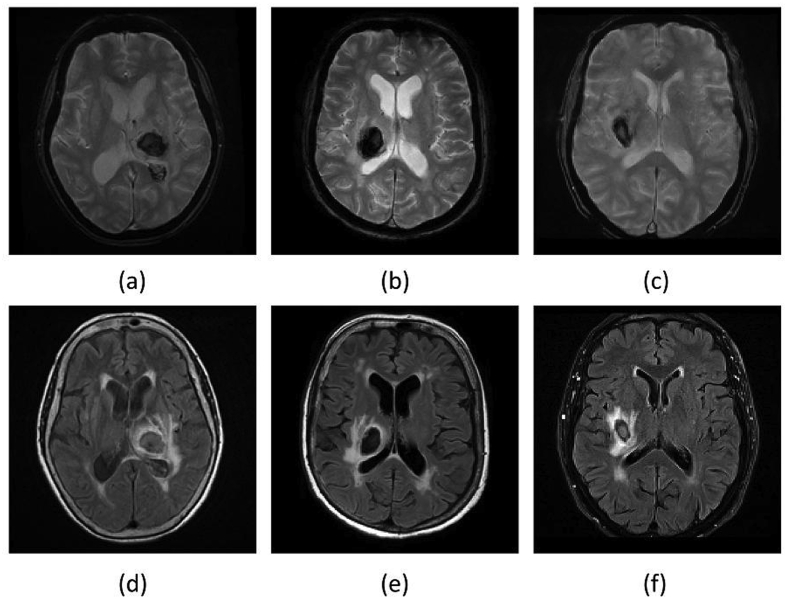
Late subacute phase MR sequences of three different SICH patients. (a)–(c): T2* GRE. (d)–(f): FLAIR. Note that on FLAIR imaging the haematoma can be completely iso-intense to grey matter, completely hypo-intense, or hypo-intense with an iso-intense centre. On the contrary, the haematomas tend to consistently appear hypo-intense in the T2* GRE images, albeit with a possible small iso-intense centre.
